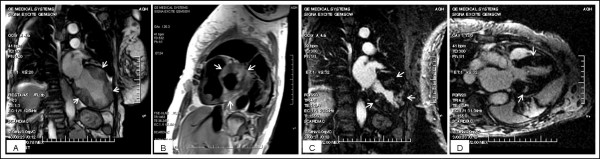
A) SSFP cine still frame demonstrates nodular appearance of the myocardium (arrows) with slightly bright signal compared to normal myocardium. Also, the endocardial border is irregular. B) T2-weighted image demonstrating bright nodular melanoma deposits (arrows) within the myocardium. C) 5 min post-contrast there is extensive patchy but discrete areas of myocardial enhancement (arrows) correlating with the nodular appearance of myocardium in 4A. D) left ventricular outflow tract view demonstrating patchy enhancement in the interventricular septum and posterior wall (arrows).
